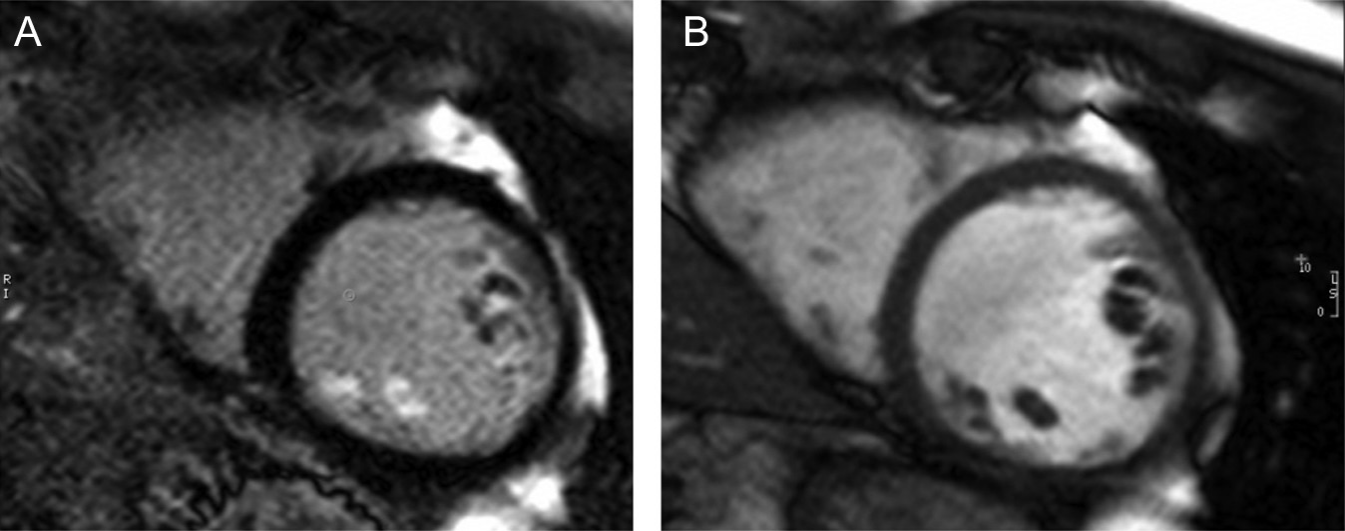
Late gadolinium enhancement of papillary muscle on cardiac MRI (A) and the corresponding cine short axis slice (B) in a patient with primary degenerative MR and normal LVEF.
